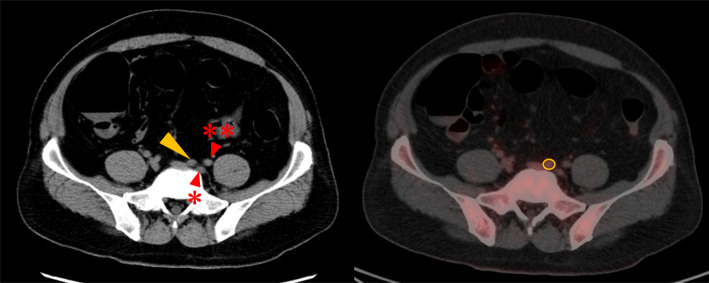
Multidetector computed tomography detecting the largest short axis of the lymph node in the left lateral lymph node region (left image), and measurement of SUVmax using region of interest on PET-CT (right image). Internal iliac artery (red arrow *), external iliac artery (red arrow ), the lateral lymph node of internal iliac region #263 (yellow arrow), the region of interest (yellow circle)
